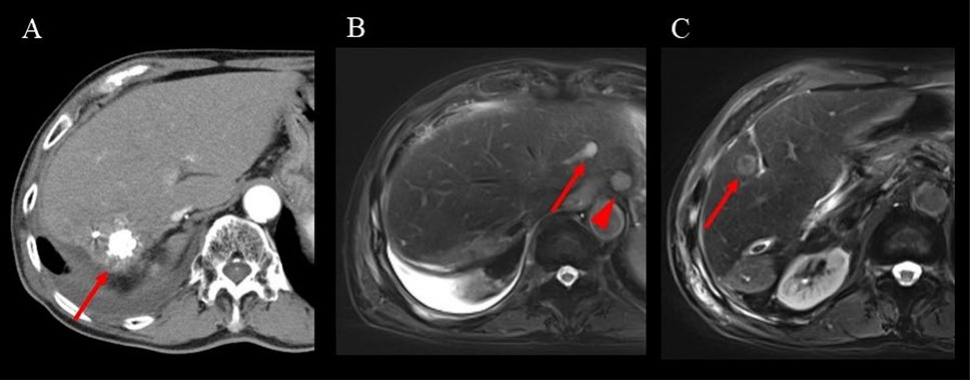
Contrast-enhanced CT after second C-ion RT and superparamagnetic iron oxide (SPIO)-enhanced MRI after all treatments. A Arterial phase CT 5 months after second C-ion RT, the red arrow shows the disappearance of the contrast effect. B, C Hepatocyte phase MRI 60 months after initial C-ion RT, red arrows show intrahepatic lesions in segments 2 and 5. The red triangle shows lymph node metastasis. All lesions were treated with TACE
